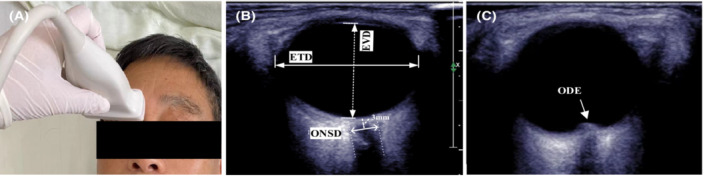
(A) Optic nerve ultrasound examination; (B) Measurement of ONSD, ETD, and EVD; An axial (transverse) view of the globe anteriorly, reveals the retrobulbar region with the optic nerve sheath (ONS) visible posteriorly. The optic nerve is visible within the ONS. (C) Measurement of ODH. ETD, eyeball transverse diameter; EVD, eyeball vertical diameter; ODE, optic disc edema; ONSD, optic nerve sheath diameter.
